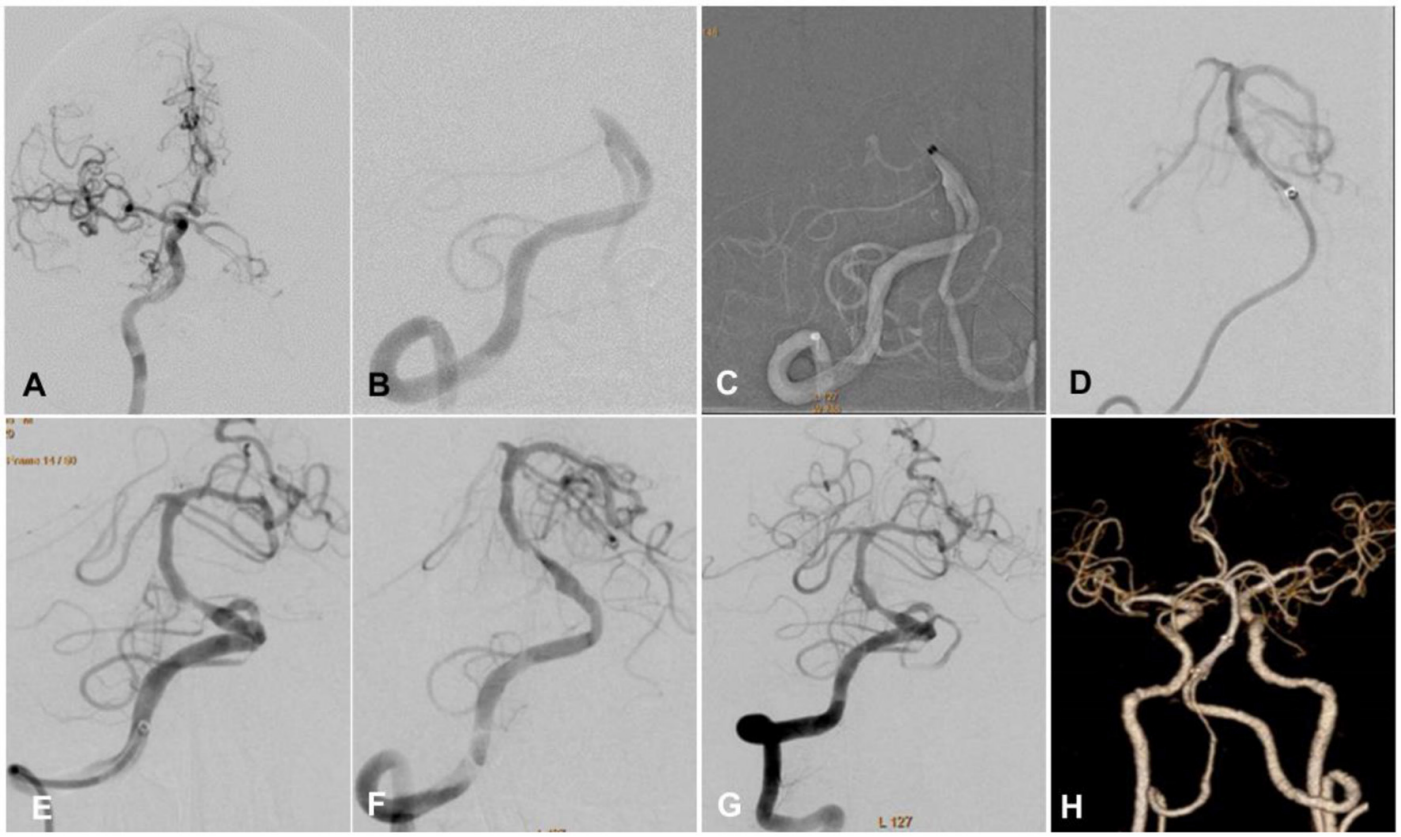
A male patient in his 60s had paroxysmal dysphasia, left limb weakness for 1 h, and coma for 30 min. The NHISS score was 19. (A) Cerebral digital subtraction angiography showed lateral circulation and basilar artery tip, suggesting intracranial atherosclerotic stenosis-related large vessel occlusion (ICAS-LVO). (B) The right vertebral artery angiography demonstrated occlusion of the initial segment of the basilar artery. (C) A REACT68 suction catheter was navigated to the basilar artery proximal to the occlusion, and after 30 s of negative pressure suction *in situ*, the retrograde blood flow in the catheter suddenly recovered. (D) Angiography confirmed that the anterior blood flow of the basilar artery returned to normal. (E) The aspiration catheter was withdrawn, and angiography revealed severe stenosis at the middle segment of the basilar artery as ICAS-LVO. (F) After 10 min of observation, the stenosis degree was increased, and the forward blood flow gradually became worse. (G) A stent was implanted after balloon pre-dilation, with the blood flow reaching mTICI grade 3. (H) Computed tomography angiography of the head and neck was performed 3 days after operation, which indicated that the stent was unobstructed, and the basilar artery forward blood flow was stable. Seven days after operation, the patient was discharged, with the NIHSS score of 1.
